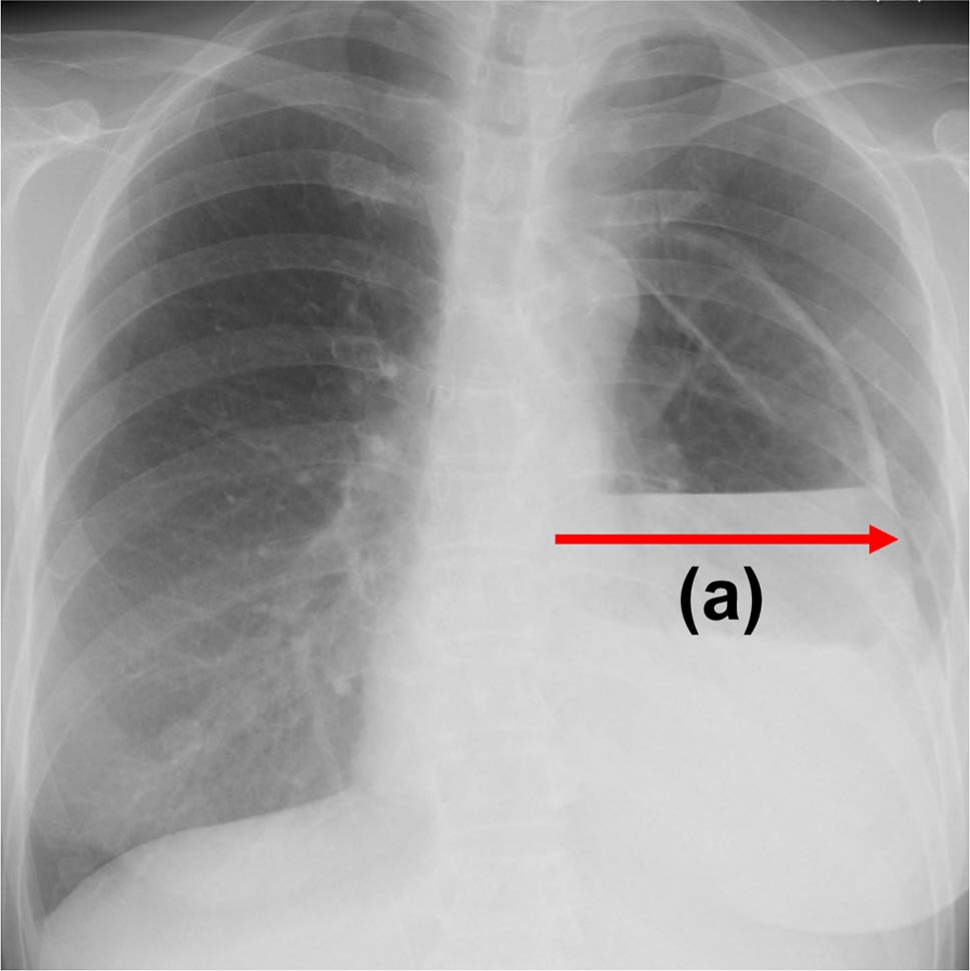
Cases in which the distance from the lateral edge of vertebra to the edge of the dilated gastric tube (a) was ≥ 5 cm on plain chest radiographs were defined as intrathoracic herniation of the gastric tube-positive
